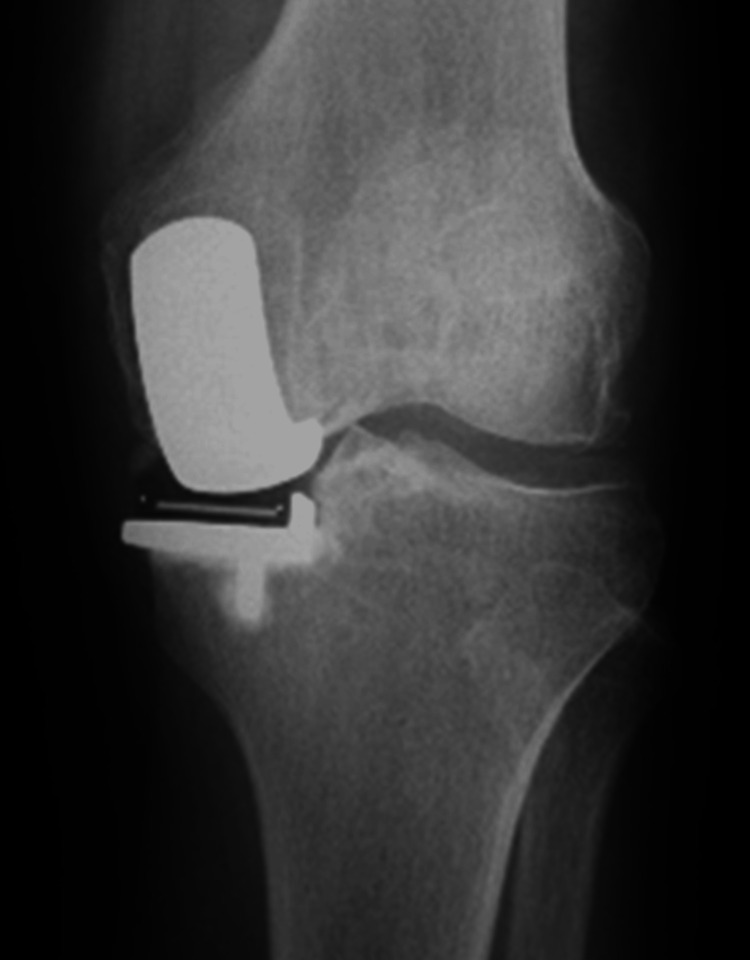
Postoperative radiography
